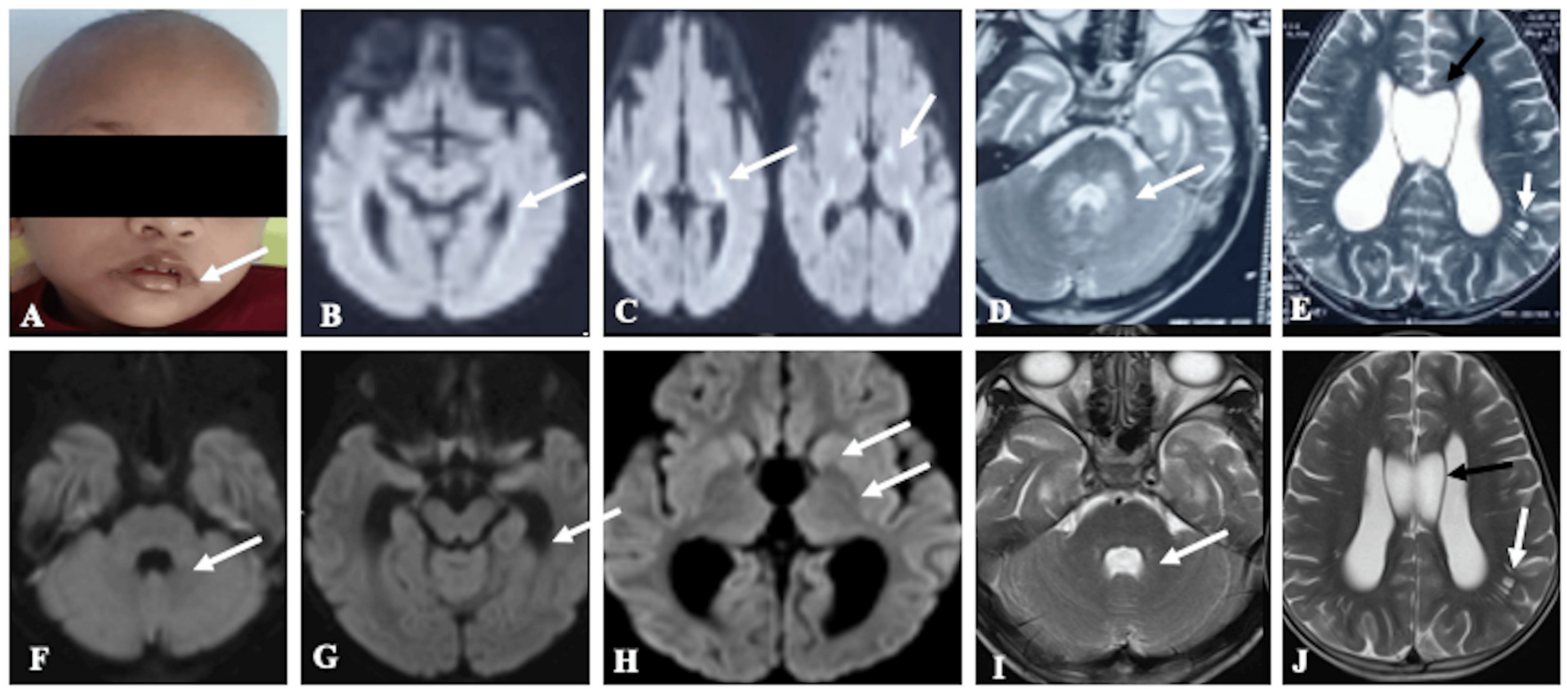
Clinical and magnetic resonance imaging (MRI) image of the patient with biotinidase deficiency Clinical photograph (A) of the patient showed alopecia with cheilitis (white arrow). The MRI image (axial DWI image) (B,C) showed relatively symmetrical restricted diffusion in the bilateral peritrigonal white matter (white arrow in B), bilateral genu, and posterior limbs of internal capsules (white arrows in C). The axial T2 image at the level of the pons (D) showed T2 hyperintensity surrounding the fourth ventricle in the pons, both middle cerebellar peduncles, and the dentate nuclei (white arrow). The axial T2 image at the level of the lateral ventricle (E) showed the cavum septum pellucidum (black arrow) and dilated Virchow-Robbins space (VRS) (white arrow). Follow-up MRI axial diffusion (F-H) and axial T2 (I,J) after two weeks of biotin supplement showed complete resolution of restricted diffusion (white arrows in F-H) and T2 hyperintensities (white arrow in I) in the corresponding regions with persistent cavum septum pellucidum (black arrow in J) and dilated VRS (white arrow in J).
